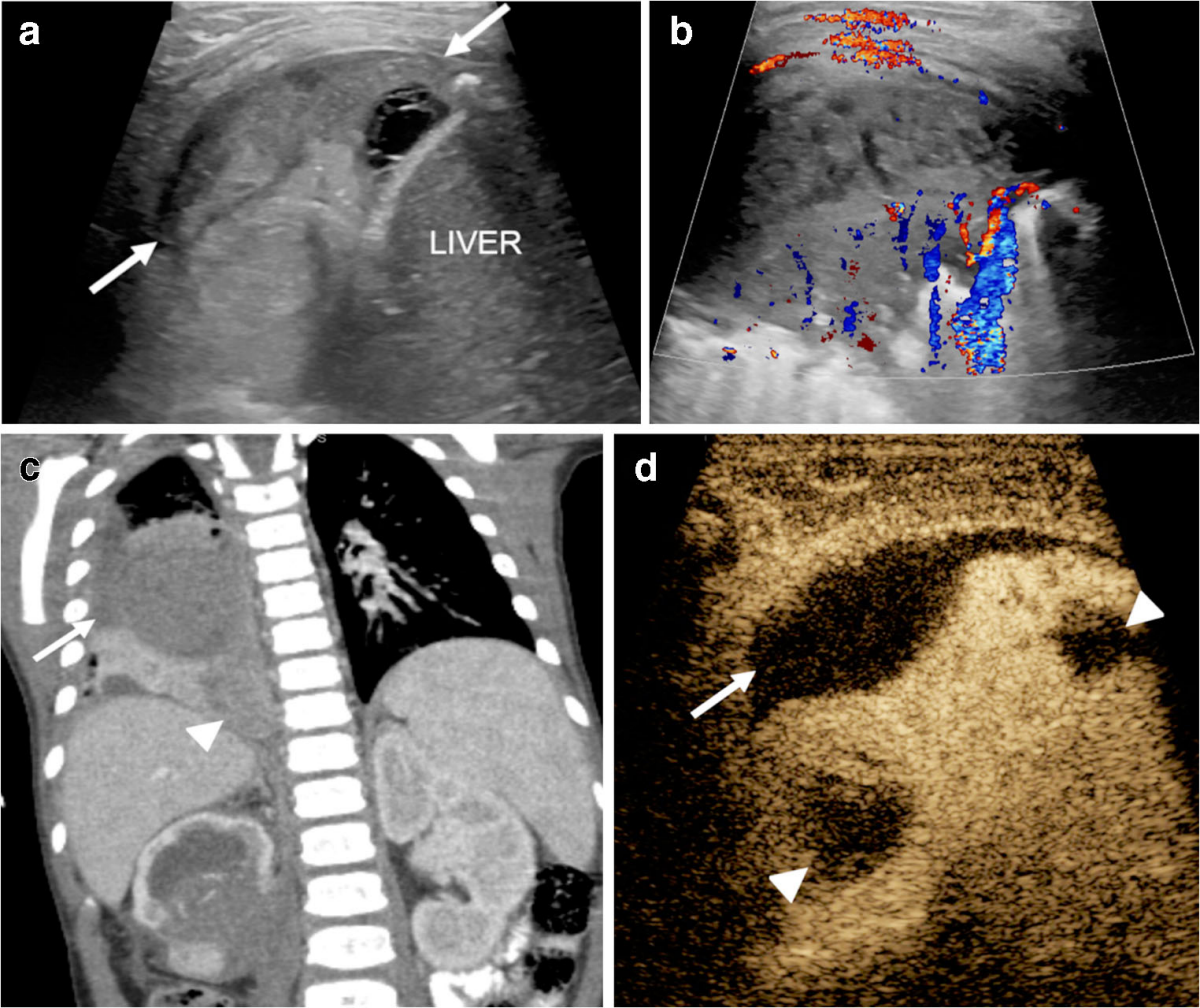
Imaging in a 4.5-year-old girl with history of rhabdoid tumor in the right kidney and lung and pleural metastases. a Coronal gray-scale US, transverse view through the right lung base posteriorly, shows a heterogeneous appearance of the right lung base (*arrows*). b Coronal color Doppler US shows some blood flow signals in the posterior aspect of the right lung base. c Contrast-enhanced chest CT, coronal reformat. The primary mass in the right kidney is demonstrated at the level of the renal pelvis extending into the proximal right ureter. Loculated low-density pleural fluid (*arrow*) is noted. There is enhancing atelectasis in the right lower lobe with a more discrete round heterogeneously hypoattenuating focus inferomedially (*arrowhead*), corresponding to lung metastasis. d Contrast-enhanced US, coronal plane. Corresponding to the CT findings, there is heterogeneous enhancement of the right lung with multifocal areas of non-enhancement or hypoenhancement (*arrowheads*) corresponding to necrosis or hemorrhage of the tumor deposits. Other areas of non-enhancement are suspicious for hemorrhagic pleural fluid from tumoral hemorrhage (*arrow*)
